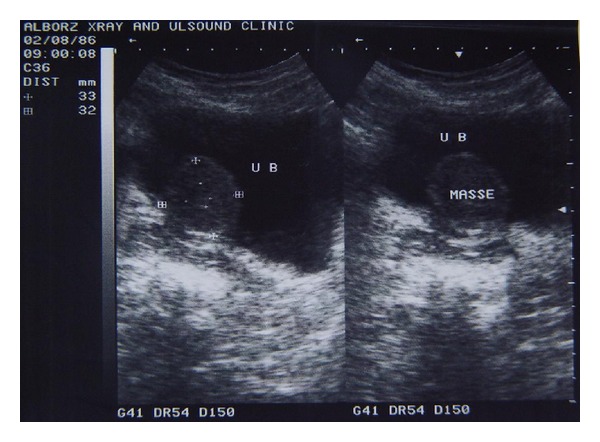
A heteroechoic round mass was revealed in ultrasonography.
